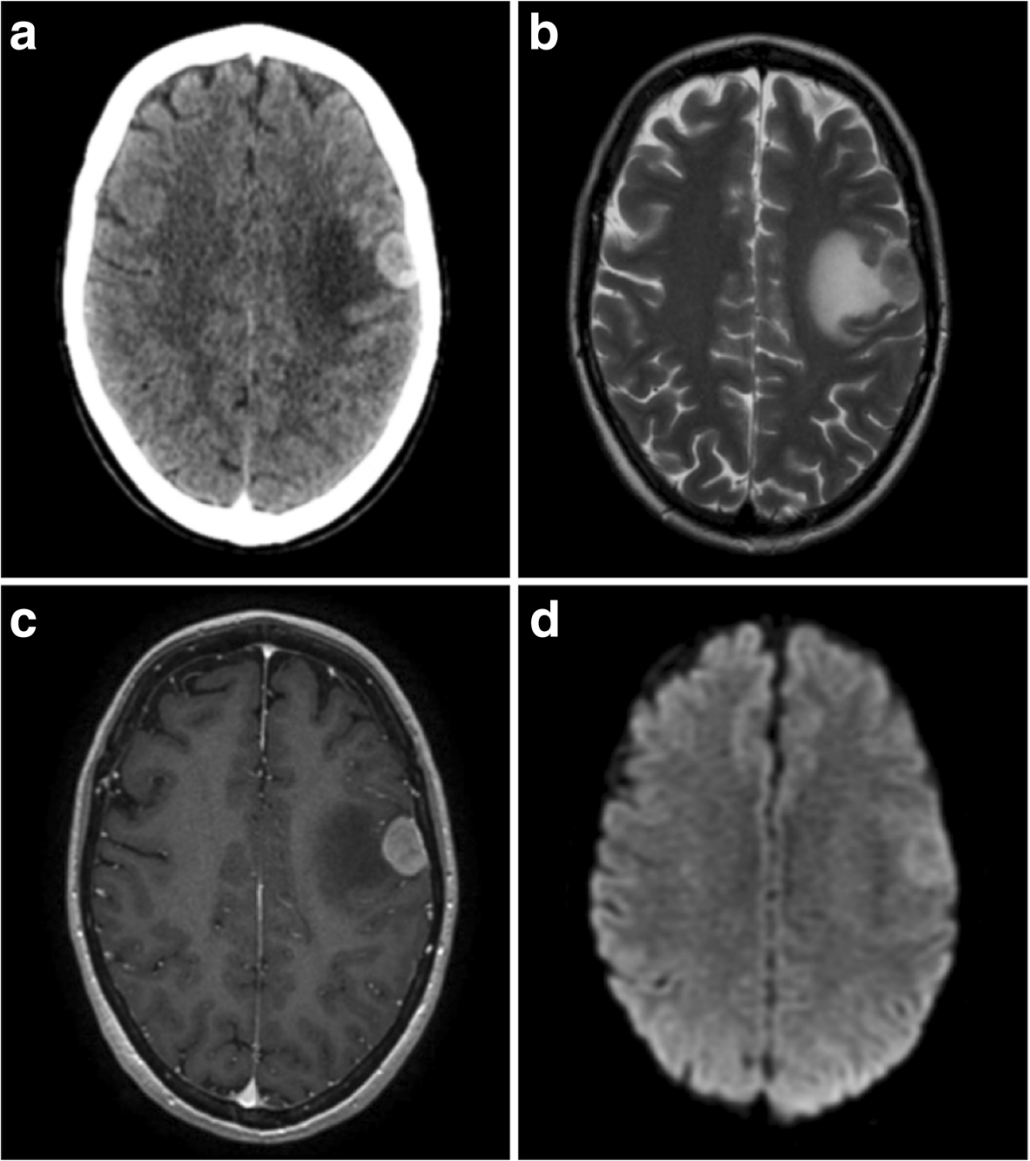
Breast cancer metastasis. a Post-contrast axial CT showing a strongly enhancing extra-axial lesion with a wide dural base and associated vasogenic oedema. There is no bony hyperostosis or erosion. b T2-weighted axial image of the same lesion which has an indistinct cortical interface suggestive of involvement. c Post-contrast axial T1-weighted MR image of the lesion with some slight heterogeneous but strong enhancement. d Diffusion-weighted imaging demonstrated intermediate restricted diffusion within the lesion
